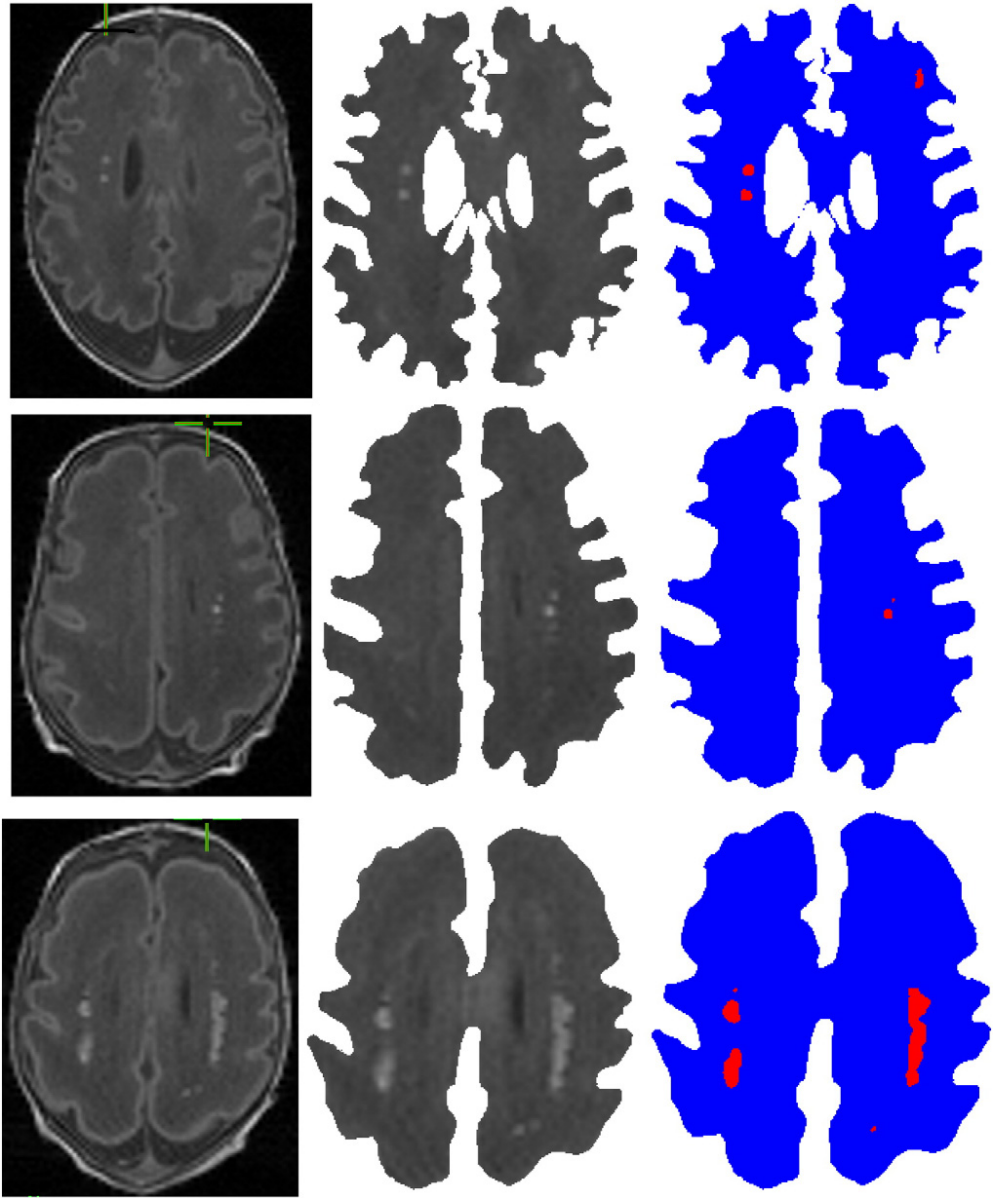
(Left) original images; (middle) white matter regions in MRI slices after pre-processing; and (right) injuries detected automatically (marked in red) by our stochastic algorithm.
